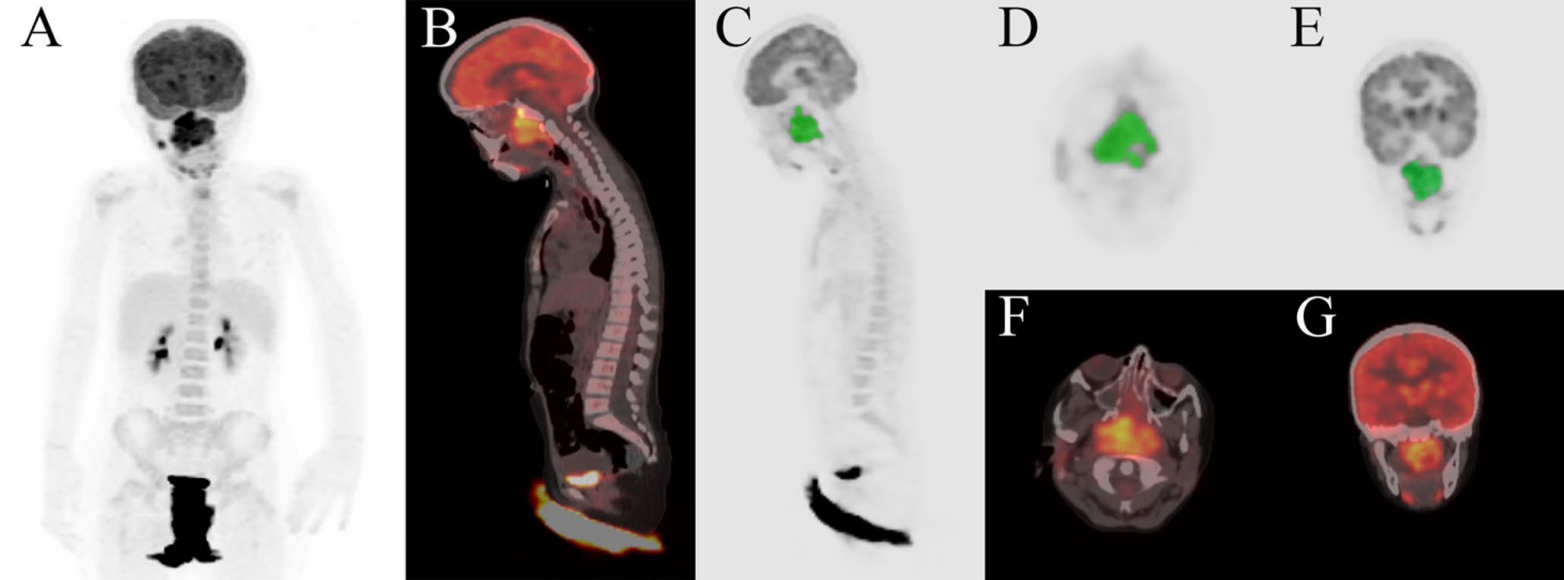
Primary tumour segmentation method based on a 40% SUV threshold in baseline ^18^F-FDG PET/CT image. A MIP image, B sagittal fusion, primary tumour segmentation in sagittal (C), axial (D), and coronal (E) PET images, axial fusion (F), and coronal fusion (G) images
